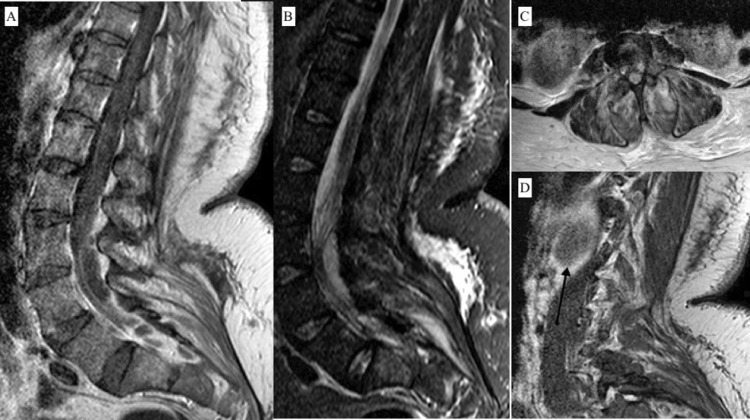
Her MRI showing a 10 x 1.3 cm dorsal epidural abscess between L2 and the distal-most thecal sac causing severe compression of the thecal sac. A. T1 image with low signal collection impinging on the thecal sac. B. T2 image with high signal collection impinging on the thecal sac. C. Collection seen posterior to the cord. D. Sagittal image of the spinal abscess.
